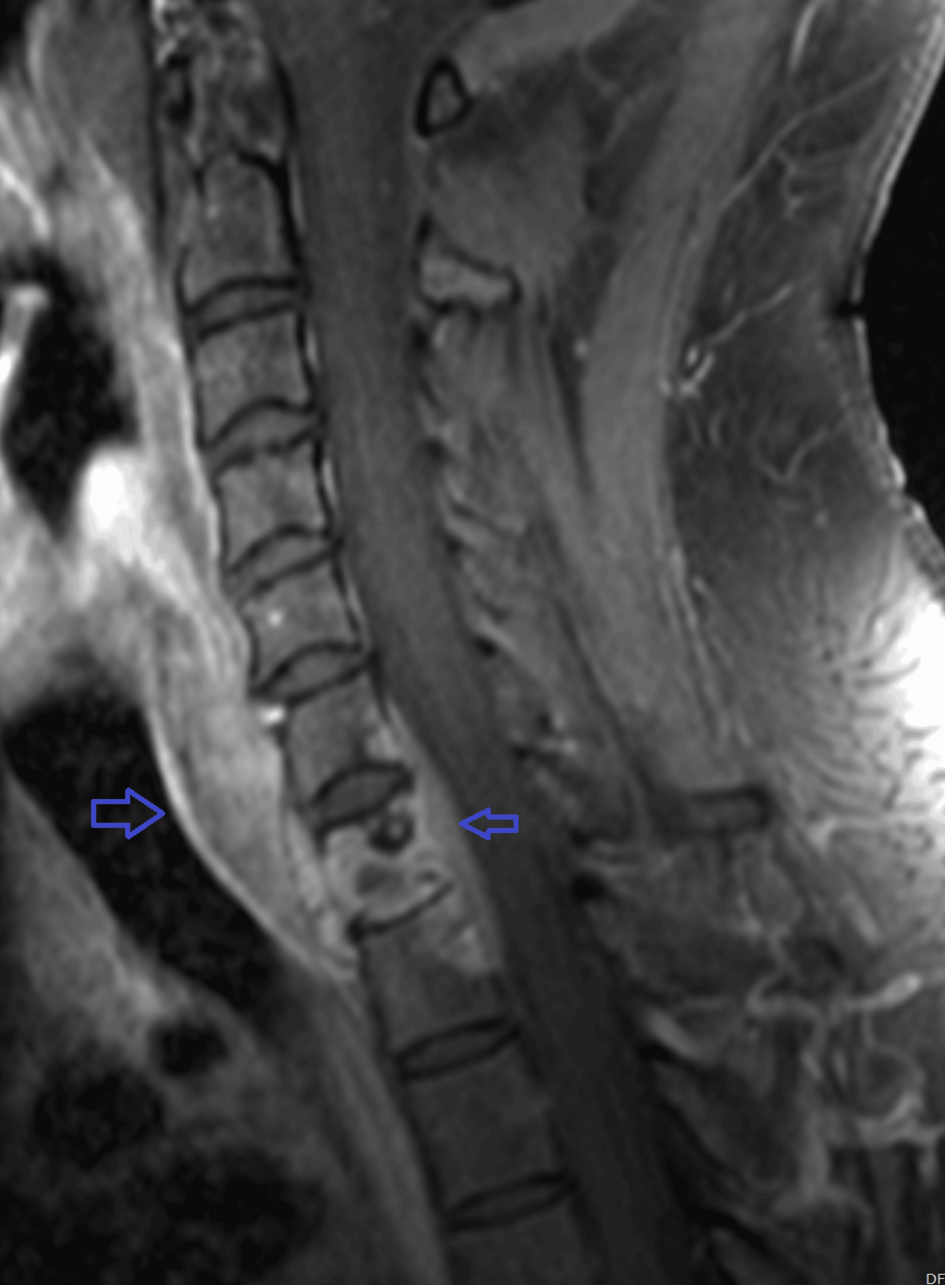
Sagittal T1 MRI showing C7 epidural abscess with involvement of the vertebral body and surrounding paravertebral soft tissues
